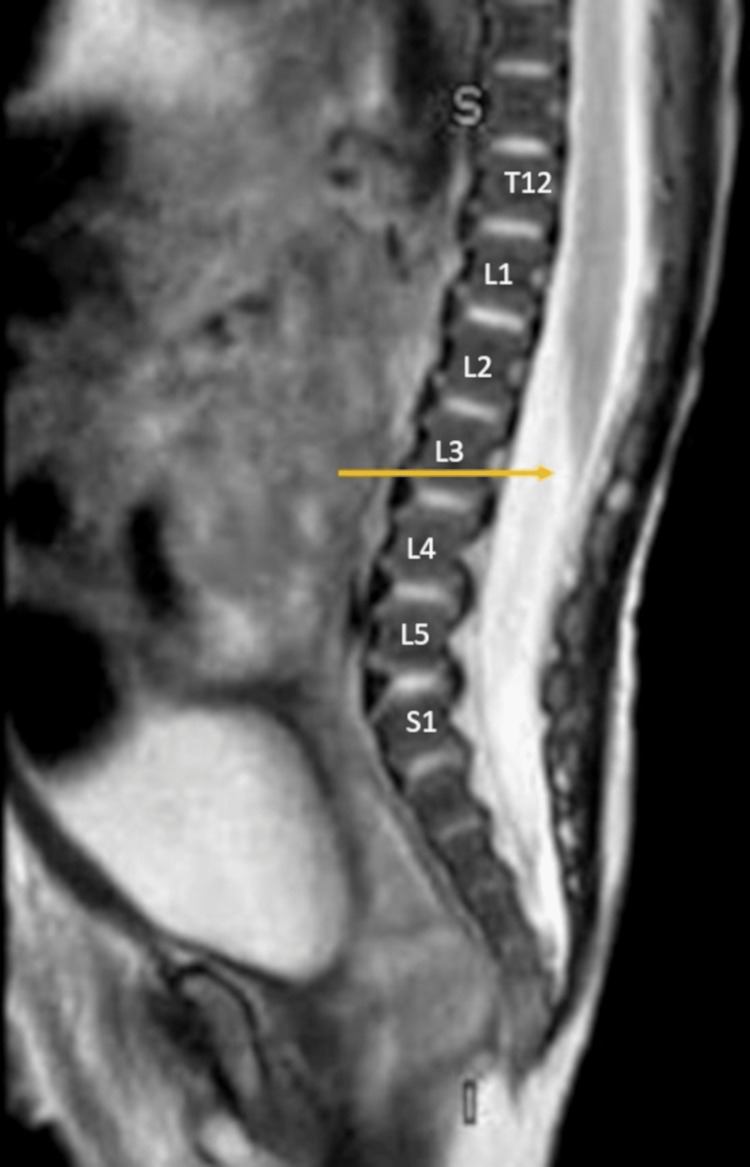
MRI Sagittal T2-weighted MRI sequence confirming borderline low-lying cord with the tip of the conus medullaris terminating at the middle of the L3 vertebrae (yellow arrow). Radiological images obtained by the authors.
